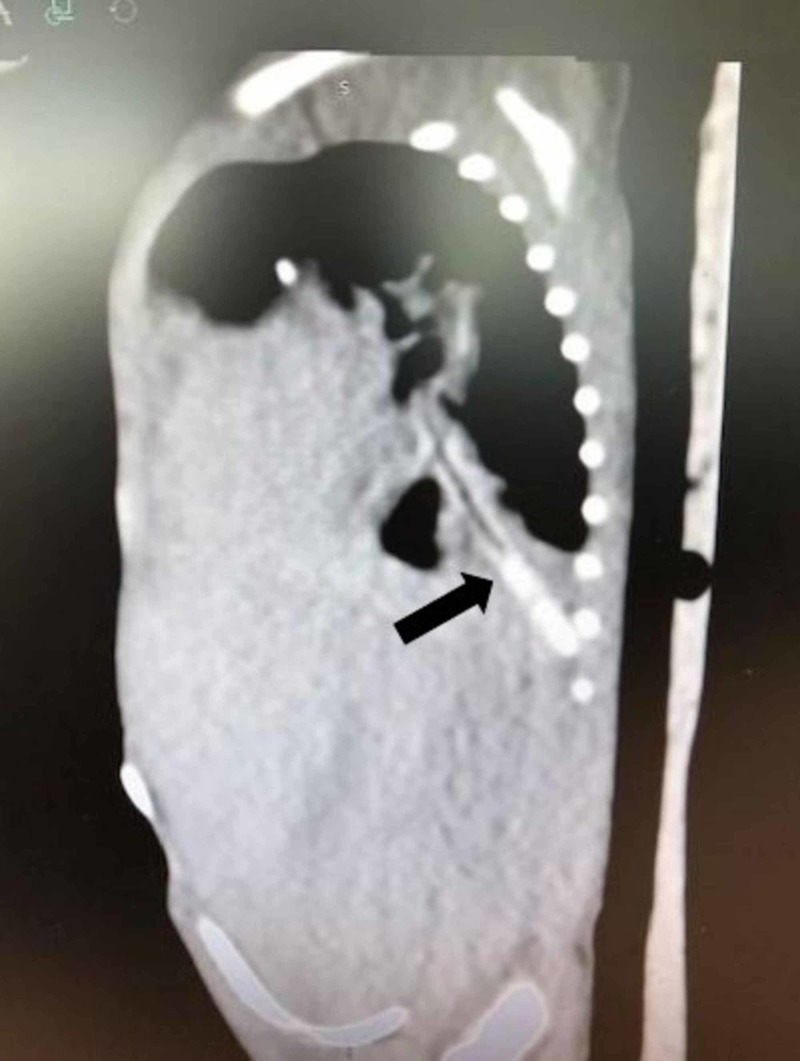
Sagittal view of CT of chest and abdomen The arrow notes the position of the stylet sheath on sagittal view of the patient's CT of the chest/abdomen. The sheath fragment extended into the most distal portion of the segmental bronchus to the posterior segment of the right lower lobe CT: computed tomography
